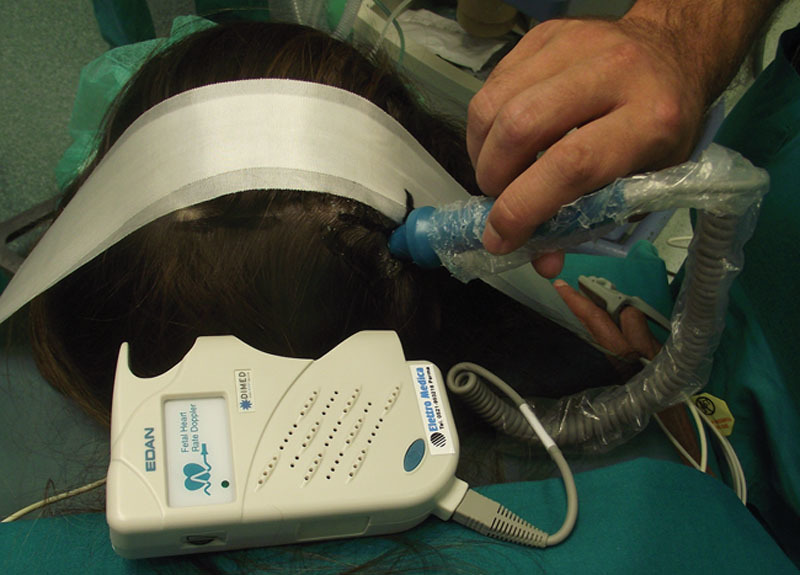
Arterial signal detected preoperatively by portable Doppler.
